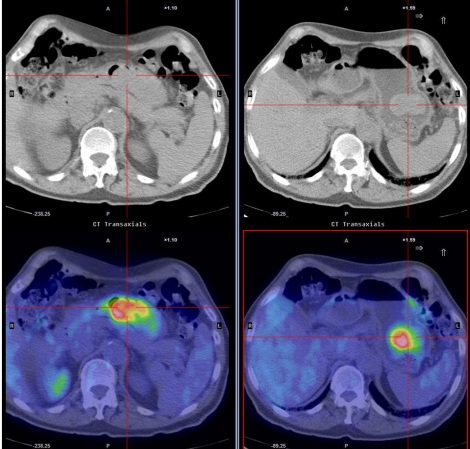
A dedicated single-bed position study acquired after whole body acquisition allows for active intervention and optimisation of CT acquisition protocols. This is particularly helpful in regions of complex anatomy like the neck and upper abdomen. These authors use this capacity for evaluation of gastric cancers, performing studies without and with gastric distension. The images on the left represent those with the stomach collapsed whereas those on the right are following gastric distension with an oral fluid load and buscopan to minimise gastric motility.
